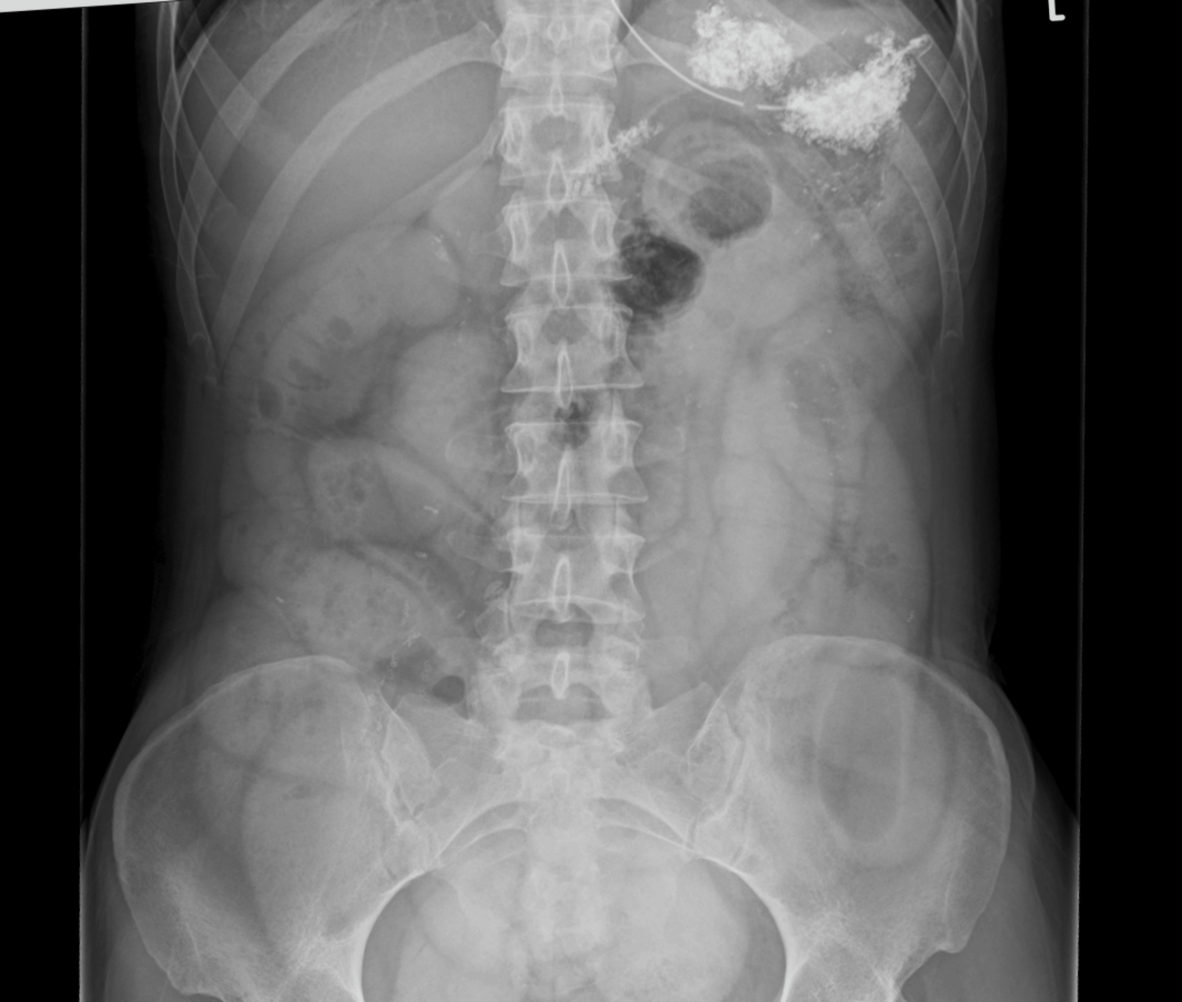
Abdominal X-ray showing diluted Gastrografin in dilated small bowel loops. It could not be confirmed if Gastrografin had passed to the colons
